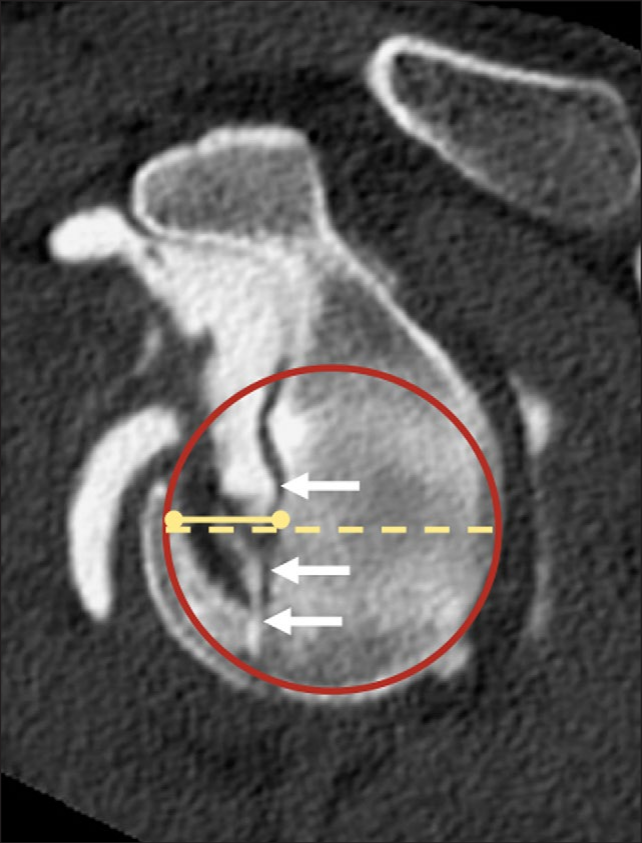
Sagittal MPR of CT arthrogram showing a quantification method for the antero-inferior glenoid bone loss in typical bony Bankart lesion (arrows). A best fit circle (red) is projected on the inferior rim of the glenoid. The width of the bone missing anteriorly (full yellow line) is divided by the diameter of the circle (dashed yellow line). A typical threshold of 20–30 percent typically indicates a glenoid augmentation procedure.
